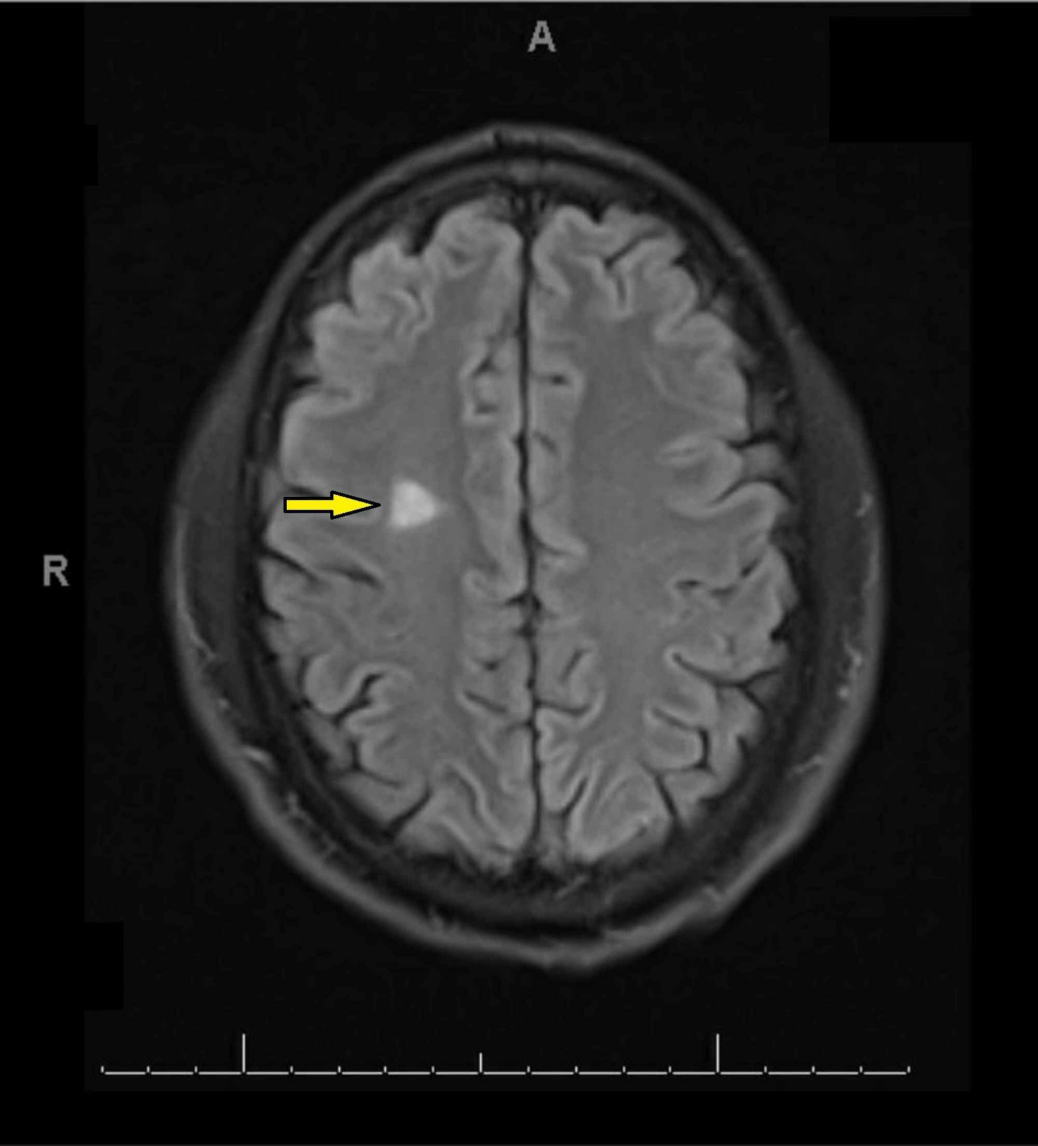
FLAIR/T2 weighted hyperintense signal foci in the right periventricular white matter on MRI.
